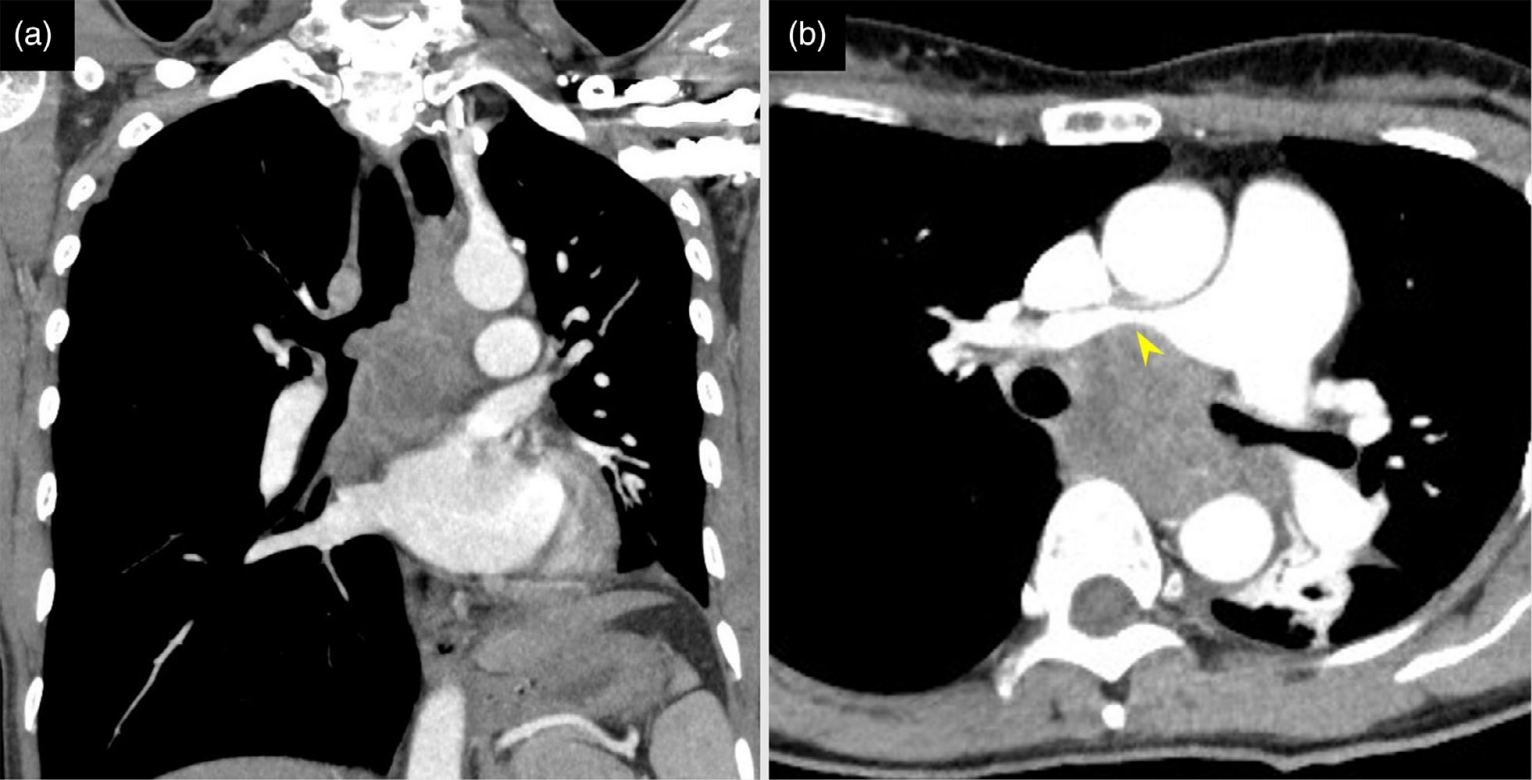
(a) Computed tomography revealed a 60 × 48 × 80 mm tumor causing left bronchial obstruction; and (b) severe suppression of the right main pulmonary artery (arrow).
